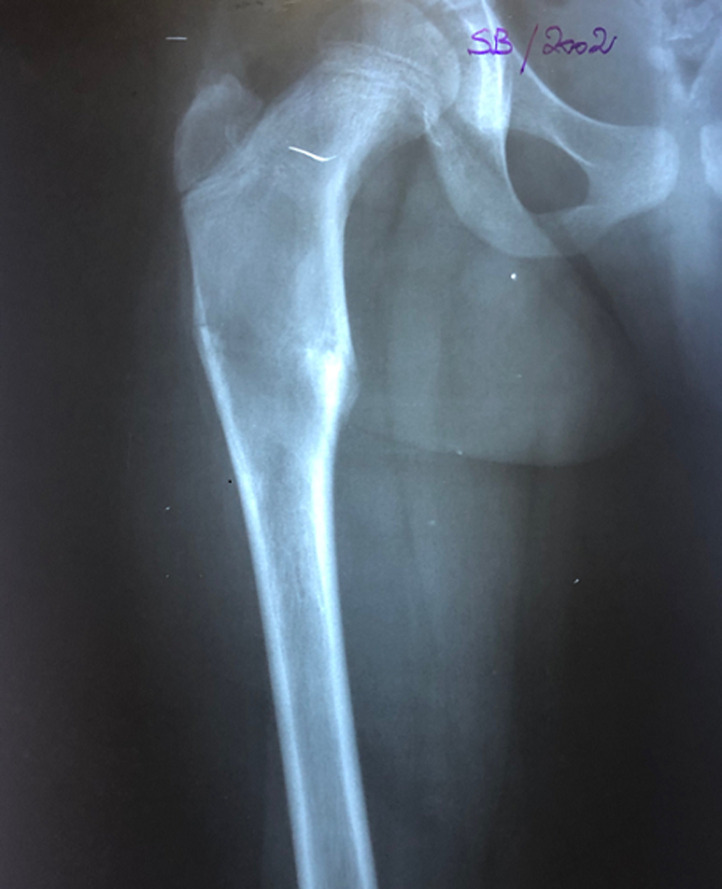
image radiographique de la lésion lytique au niveau de l´extrémité proximale du fémur dans le cadre d´une dysplasie fibreuse
